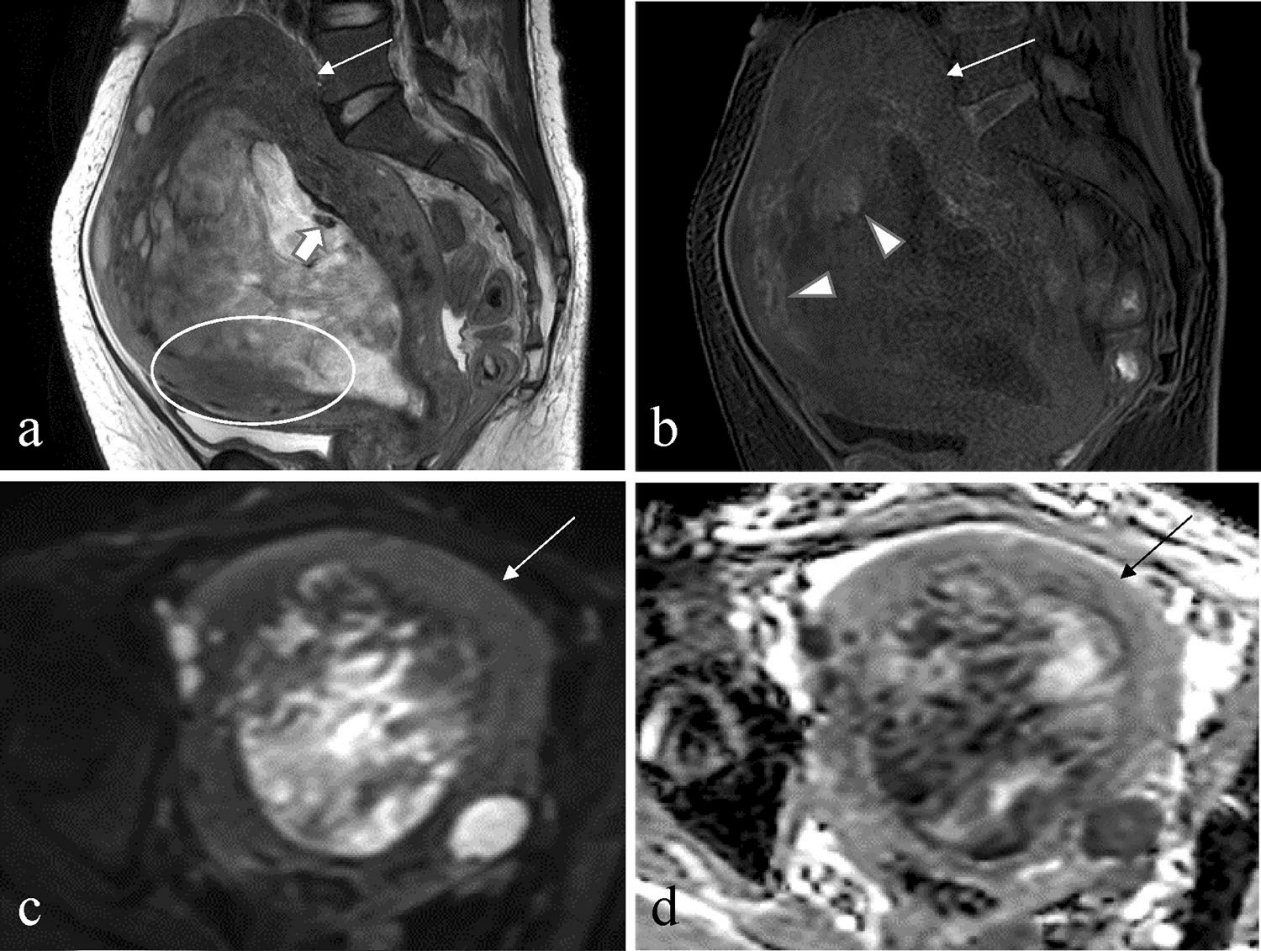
A 56-year-old woman with leiomyosarcoma with abnormal vaginal bleeding. a The sagittal T2-weighted image shows a tumor, mainly located in the uterine cavity, with an ill-defined margin (circle) and T2 dark area (arrow). The tumor shows signal heterogeneity on the T2-weighted image. b The tumor shows a T1 bright area (arrowhead) on the sagittal T1-weighted image. c On an axial diffusion-weighted image with a *b*-value of 1000 s/mm^2^, the lesion appears to have a higher signal than the myometrium (thin arrow). d The axial apparent diffusion coefficient map reveals that the solid component of leiomyosarcoma shows restricted diffusion, and the mean apparent diffusion coefficient value is 0.77 × 10^−3^ mm^2^/s
